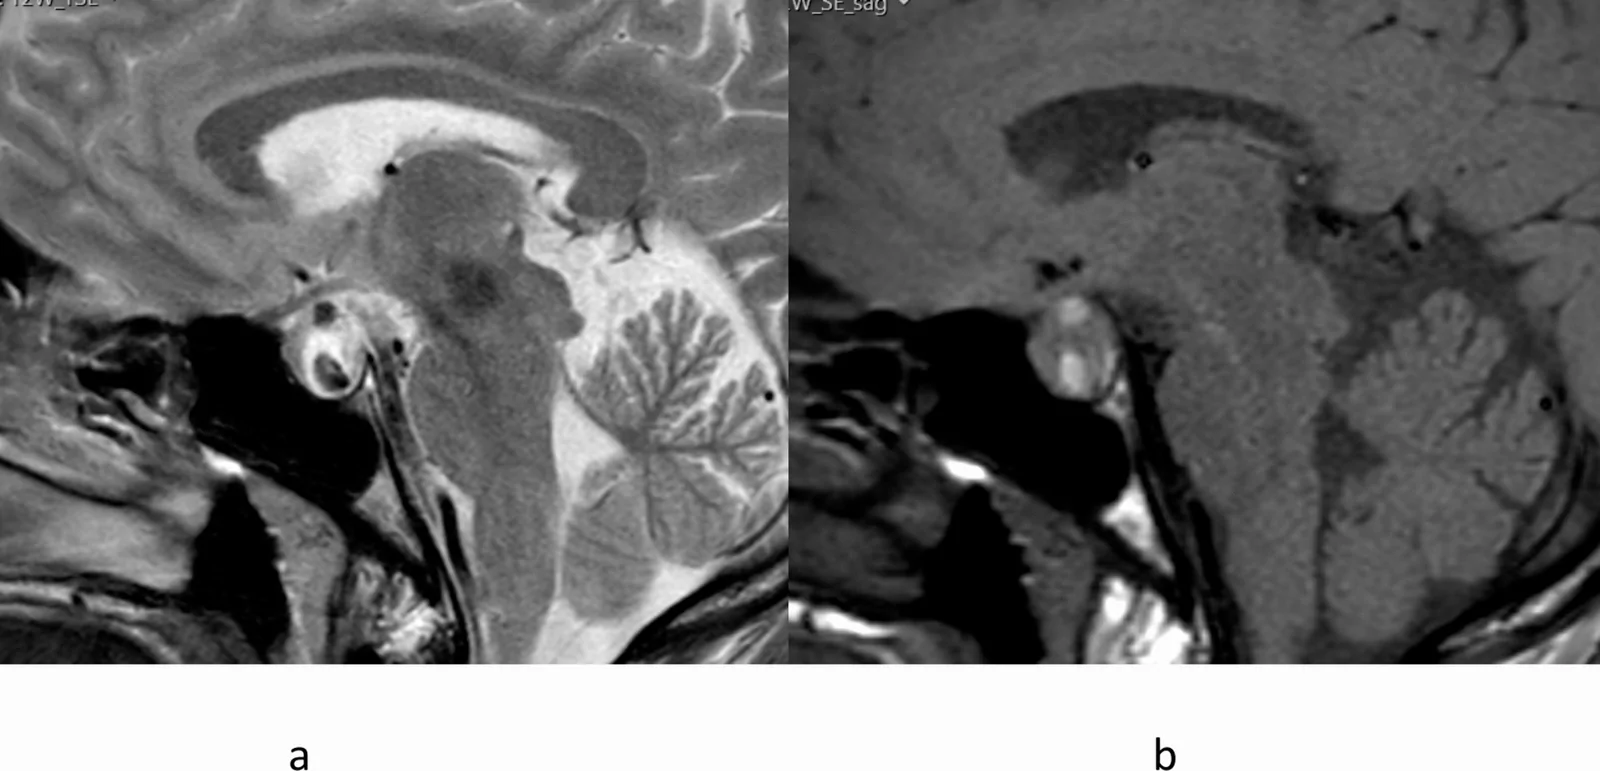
The case of a 34-year-old female with a surgically verified RCC with multiple intracystic nodules. Magnetic resonance images demonstrate an RCC with two nodules in the cyst that display a low signal intensity on the sagittal T2-weighted image (a) and high signal intensity on the sagittal T1-weighted image (b)
